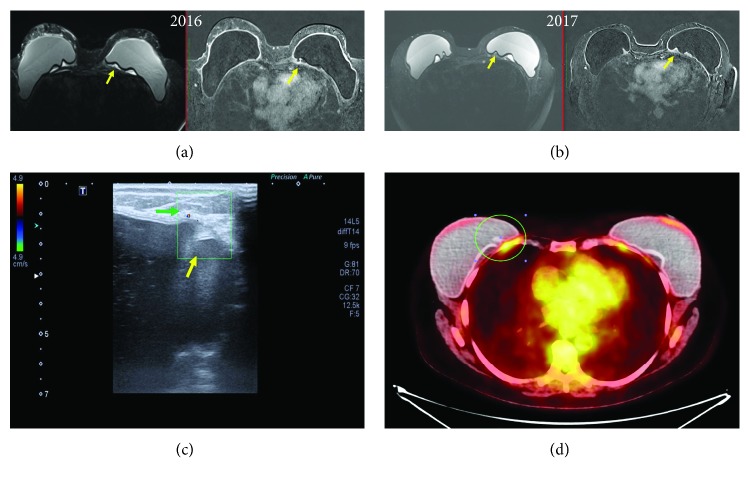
SIGBIC with extracapsular extension. 52-year-old woman with left breast stiffening. (a) T2-weighted BMRI shows an intracapsular hypersignal mass posterior to the implant (left image). After contrast, there is small enhancement of the mass (right image). (b) One year after the first scan, the patient presented an increase of mass volume and of contrast enhancement. Yellow arrows correspond to SIGBIC. Diffuse thickening of the fibrous capsule is also observed. (c) Ultrasound. Doppler scan shows an increase in vascularization at the periphery of the implant, where an intracapsular mass is observed, determining a snowstorm-type appearance. There are also changes of the inner implant echotexture. (d) PET scan. Radioisotope uptake by the mass (green circle). There is also uptake at the ticked fibrous capsule. The radiological differential diagnosis are late seroma, infectious disease, and BIA-ALCL.
